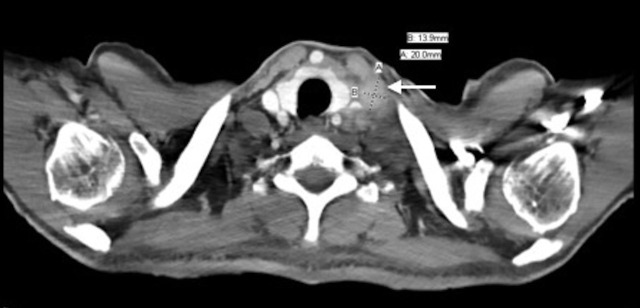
Transverse CT image taken from Patient 1 before treatment. This image shows a left supraclavicular adenopathy measuring 1.4 x 2.0 cm.
